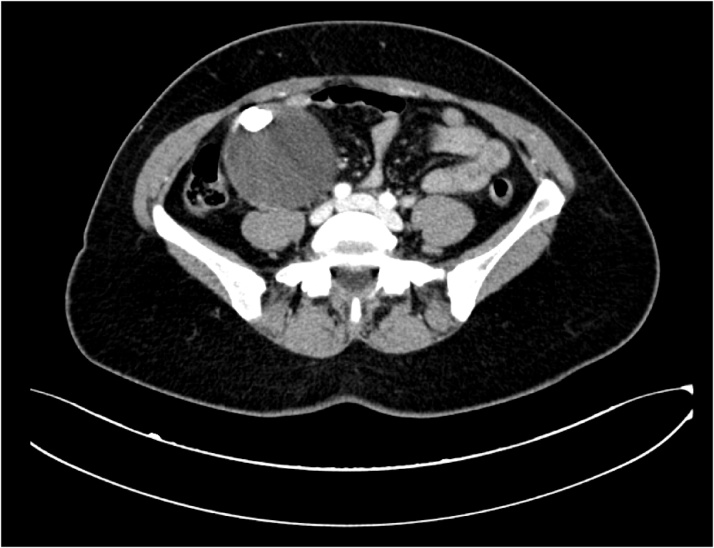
CT scan of the abdomen and pelvis, demonstrating the mass in the right abdomen.
